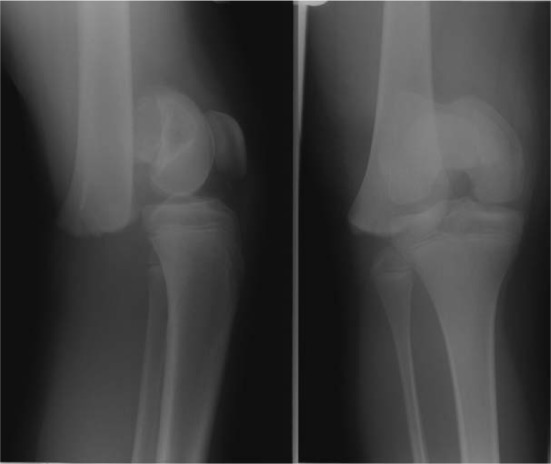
AP and lateral radiographs showing SH-1 fracture of the distal femur.
